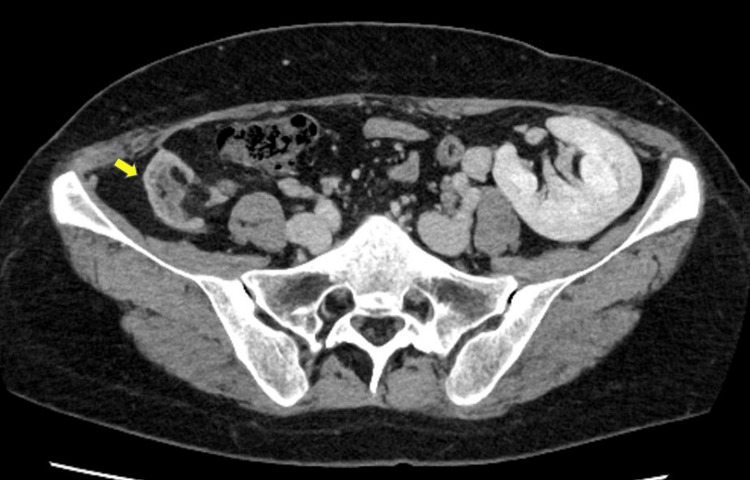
Computed tomography with contrast Computed tomography with contrast shows the bilateral transplanted kidneys in the iliac fossae. The right transplanted kidney is atrophic (arrow).
